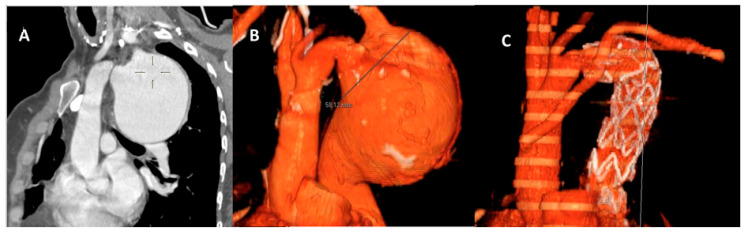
Pre- (A,B) and postoperative (C) CT Scan images showing large thoracic aneurysm involving the distal arch and descending aorta, where a surgical hybrid prosthesis is deployed in the descending aorta. Distal anastomosis is performed in zone 2 and extra-anatomic left subclavian artery bypass.
